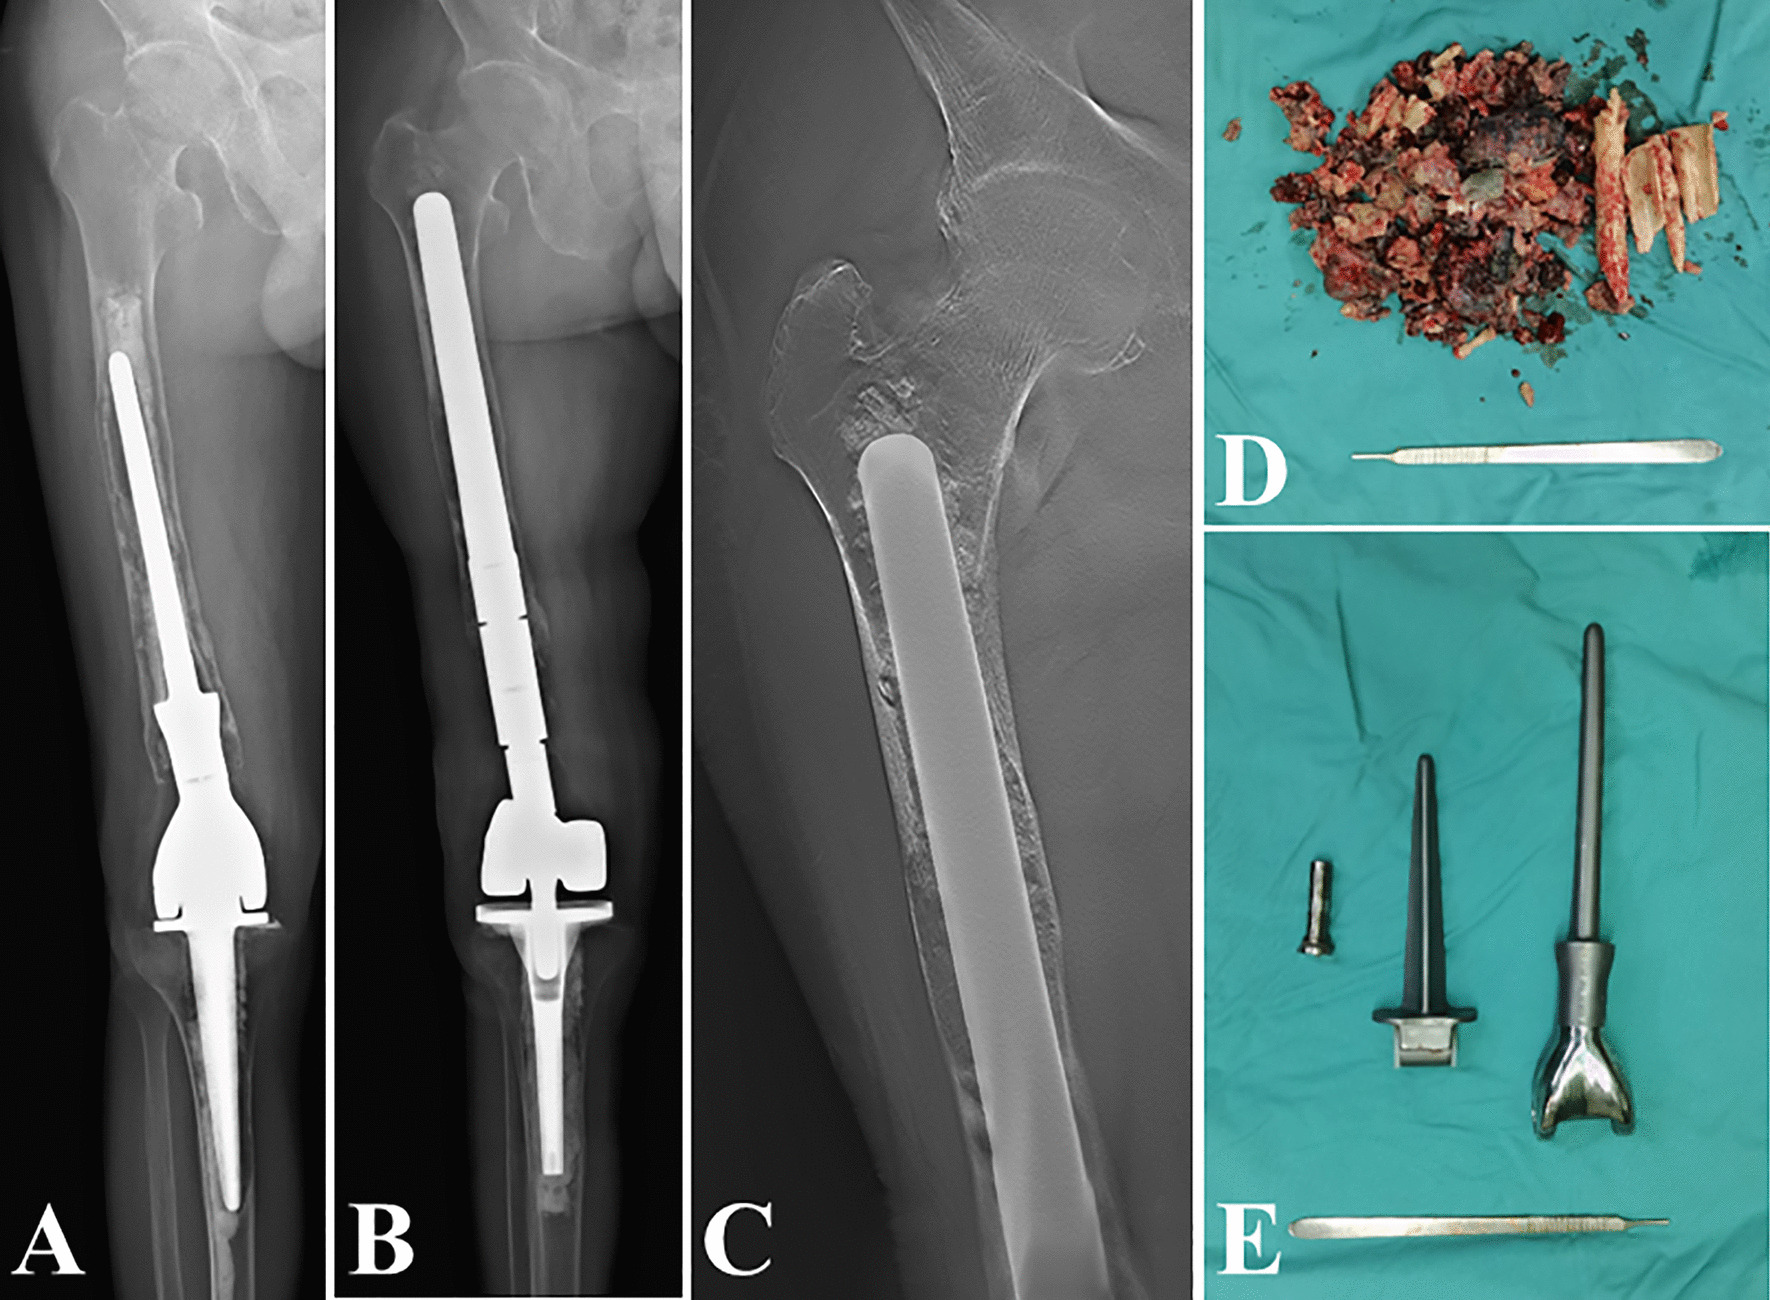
Revision of a Grade III bone loss case with “Ultra-long uncemented stem”. A, B preoperative radiographs; C the digital tomosynthesis image 6 months after revision surgery; D the surrounding hyperplastic tissue photograph; E the initial cemented endoprosthesis photograph
